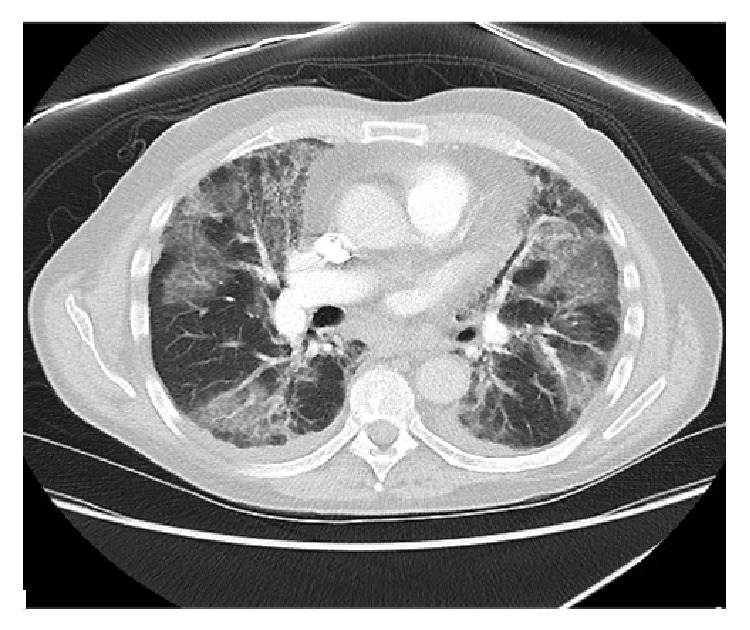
CT scan, representative cut showing b/l ground glass opacities with septal thickening from acute lung injury.
